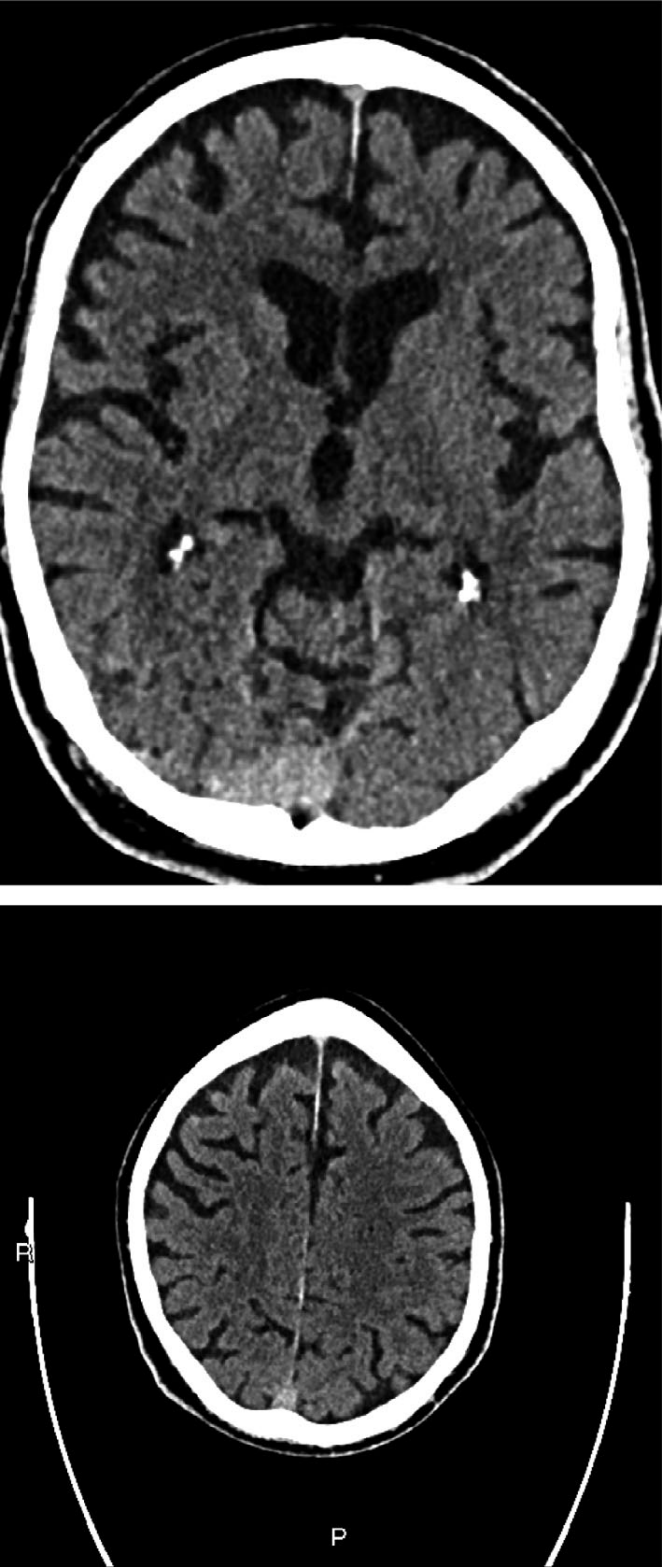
Plain CT head—there are mild chronic small vessel ischaemic changes seen. No cerebral atrophy. Basal ganglia, thalami, and brainstem structures are within normal limits. CSF drainage pathways are within normal limits. Brainstem and posterior fossa structures are within normal limits
